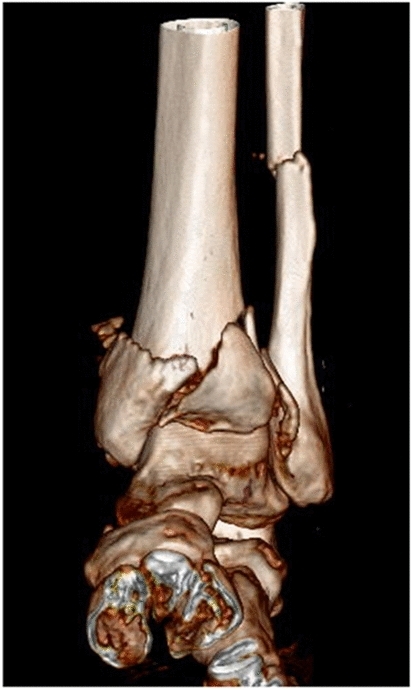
CT three-dimensional reconstructed coronal image of a comminuted distal tibia-fibula fracture of the left lower limb
